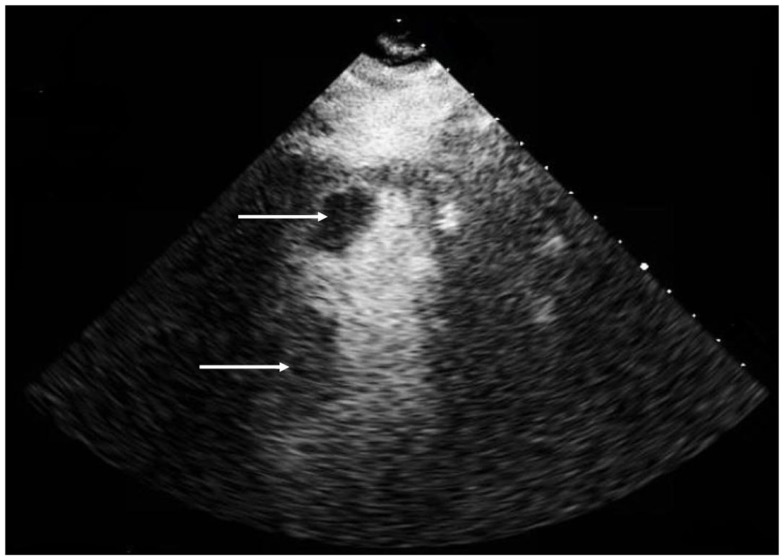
Transthoracic echocardiogram, apical 2 chamber view with contrast demonstrating a left ventricular apical thrombus (2.4 cm × 1.4 cm) (top arrow) and a second thrombus (1.6 cm × 2.2 cm) (bottom arrow) adherent to the interventricular septum.
